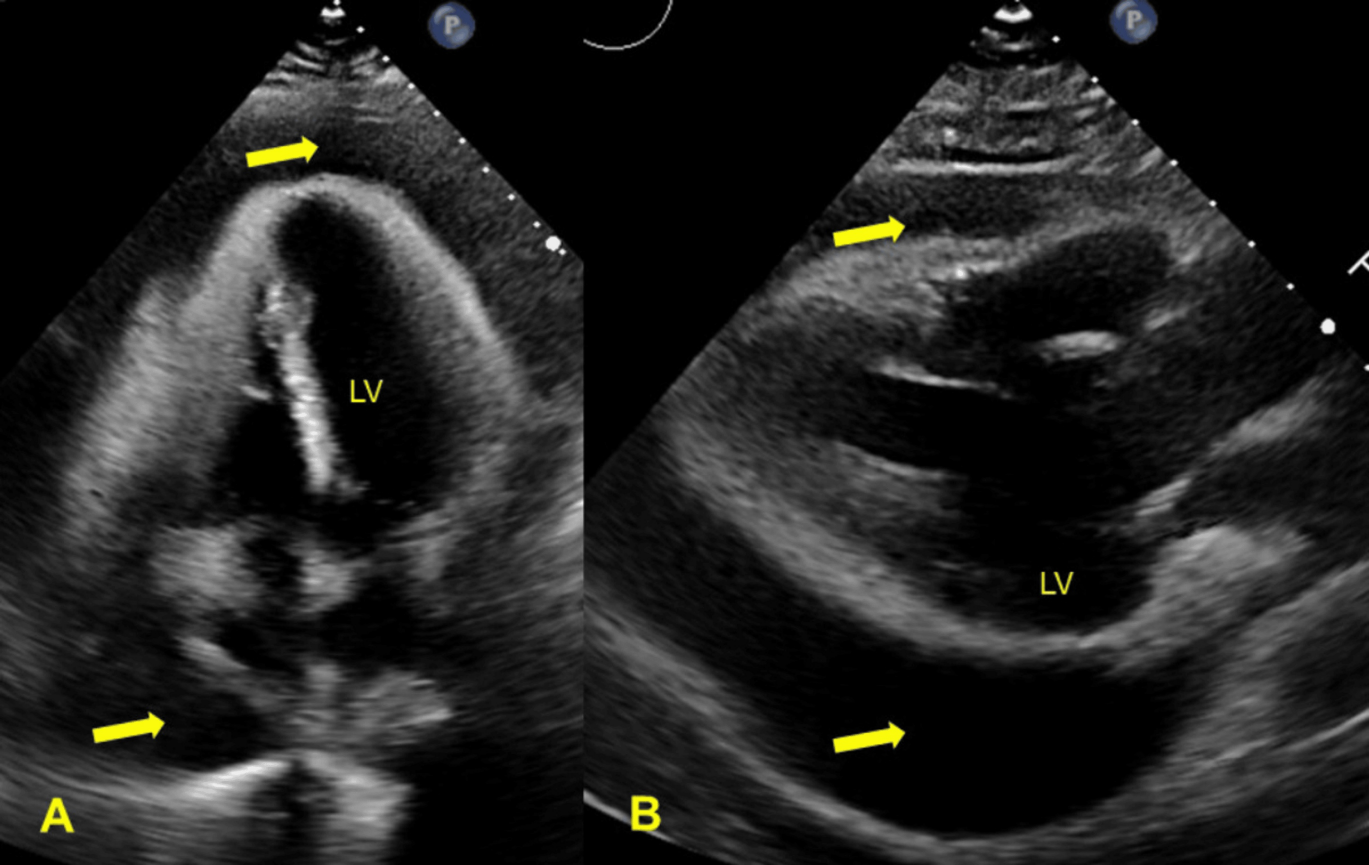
Transthoracic echocardiograms at emergent presentation (day 0) Large circumferential pericardial effusion is seen in (A) the apical four-chamber view (arrows) and (B) the parasternal long-axis view (arrows). LV: left ventricle
